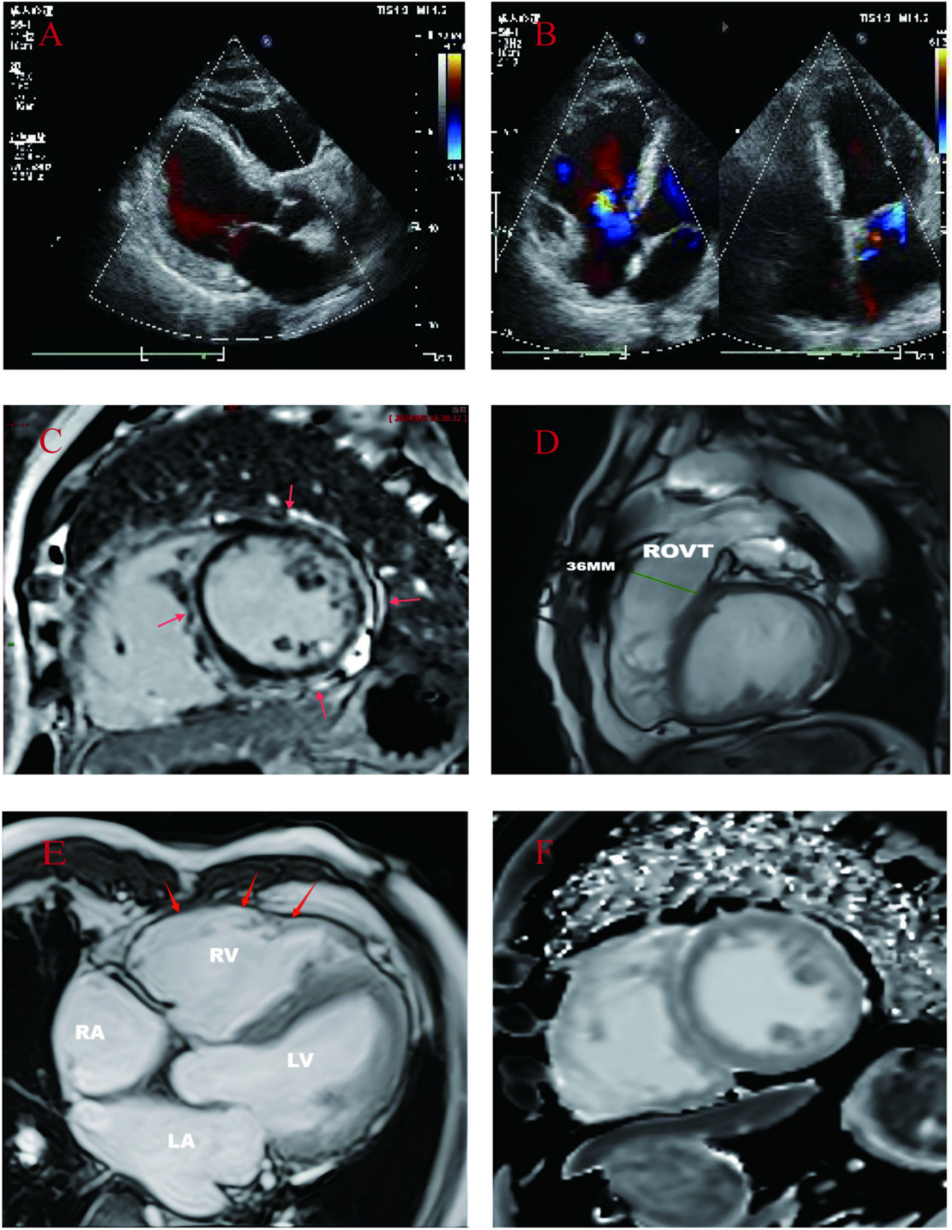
(A,B): Proband's echocardiogram: showed whole heart enlargement, overall movement weakened, left ventricular EF45% FS23%. (C–F): CMR images of probands. (C): Cardiac short-axis LGE: delayed enhancement between the free wall of the middle left ventricle and the subepicardium of the right ventricle, and delayed enhancement between the muscular walls of the interventricular septum. (D): Right ventricular outflow tract widening: 36 mm. (E): Left and right ventricular wall multiple thinning, right ventricular wall tumor (local myocardial contradictory movement). (F): Short axis native T1: increased T1 value between subepicardial left ventricular free wall and interventricular septum muscle wall.
